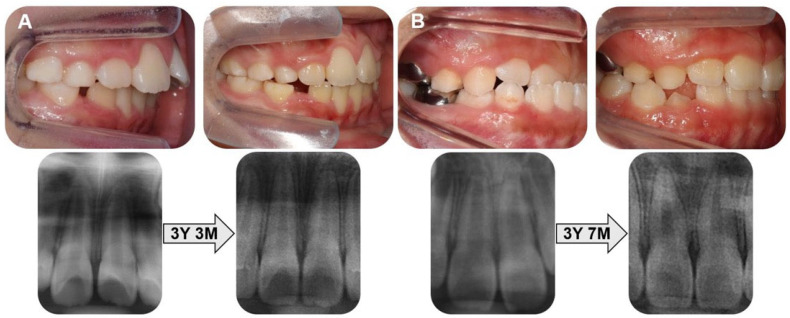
Pre- and post-treatment intraoral photographs and panoramic radiographs. (A) Patient with Class II malocclusion. (B) Patient with functional anterior crossbite.
